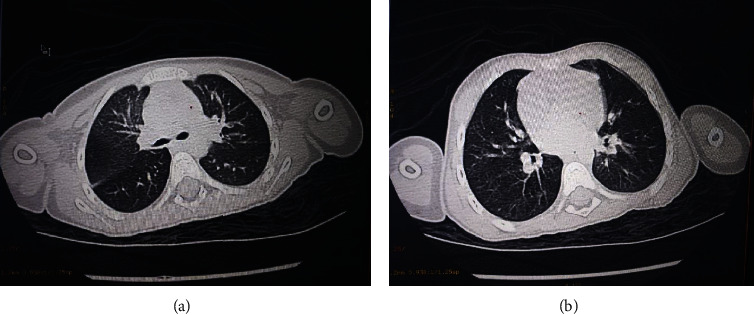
Chest CT scan showing images in favor of pneumopathy COVID-19 CO-RADS 4.
